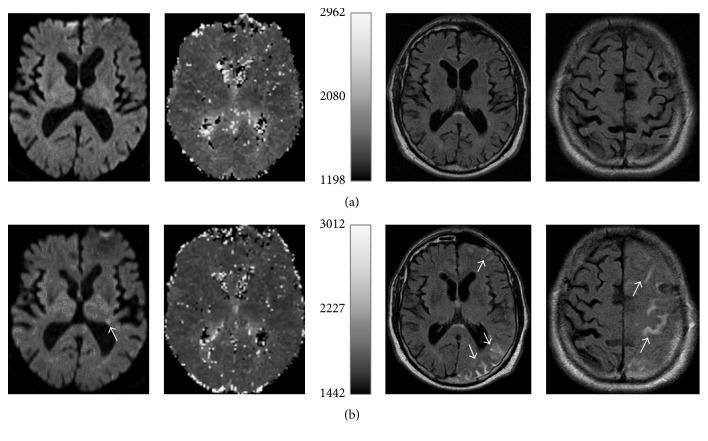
Initial (a) MRI with unremarkable diffusion-weighted imaging (DWI) and perfusion-weighted imaging (PWI) as well as FLAIR images. Follow-up MRI (b) shows small ischemic lesions (arrow) in the left MCA territory on DWI. PWI is unremarkable. FLAIR images demonstrate gadolinium enhancement in the subarachnoid space in the left MCA territory (arrows).
